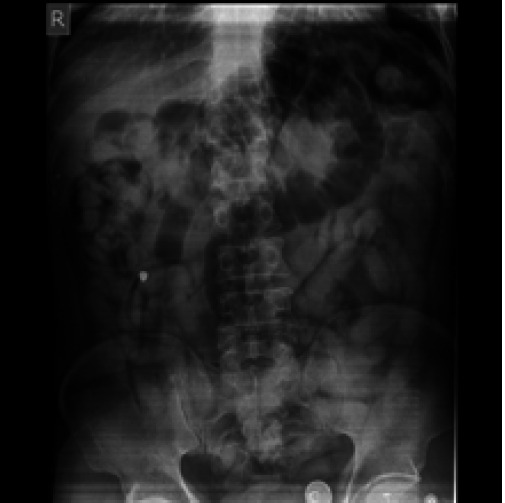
Abdominal radiograph showing multiple well circumscribed foreign bodies.
